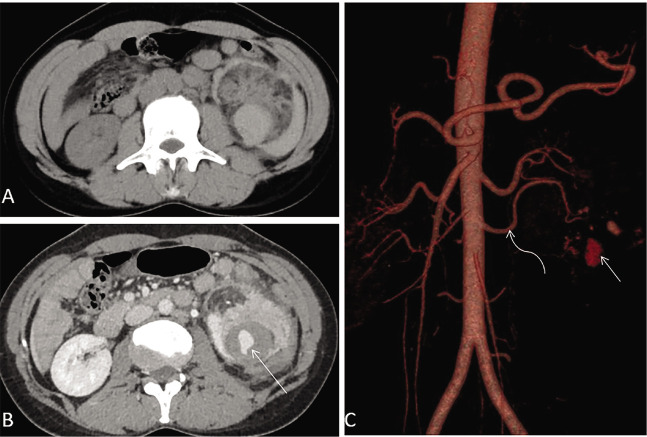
A) Noncontrast CT axial section showing heteroattenuating lesion in left kidney with intratumoral fat density and perinephric hematoma. B) CECT showing intratumoral ruptured pseudoaneurysm (straight arrow). C) Virtual 3D reconstruction showing left accessory renal artery supplying lower pole of left kidney (curved arrow).
